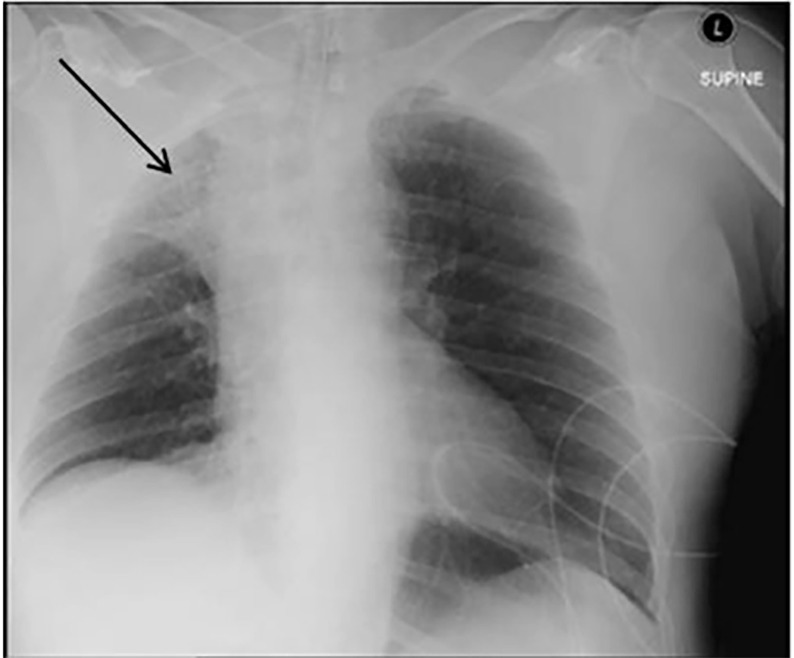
Initial chest radiograph demonstrating mediastinal widening and collapse of the right upper lobe (arrow) in patient with esophageal perforation
